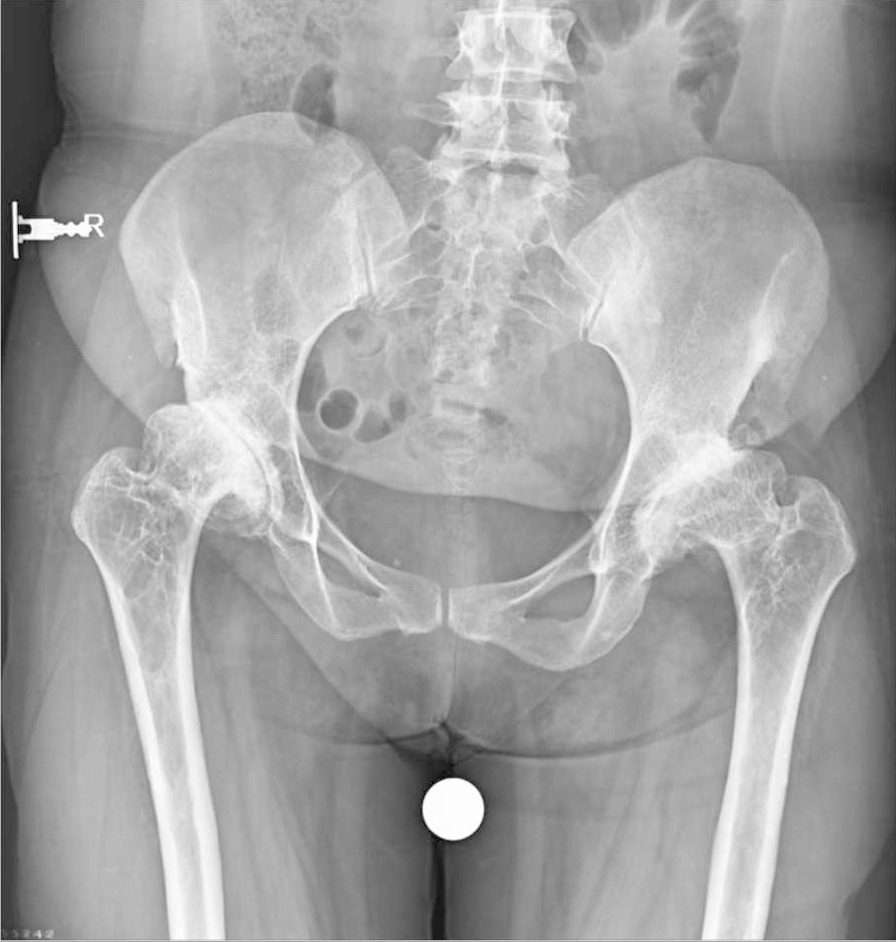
Plain hip radiograph of the patient
